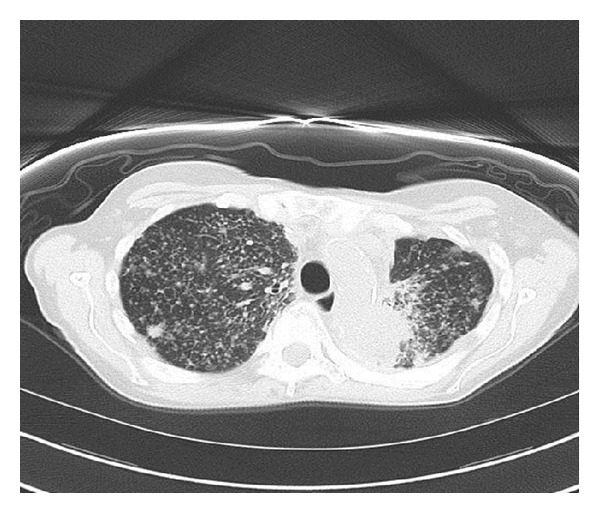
Chest CT without contrast: pulmonary nodules of varying sizes persisting throughout the lung zones, with the largest shown here in the right upper lobe.
